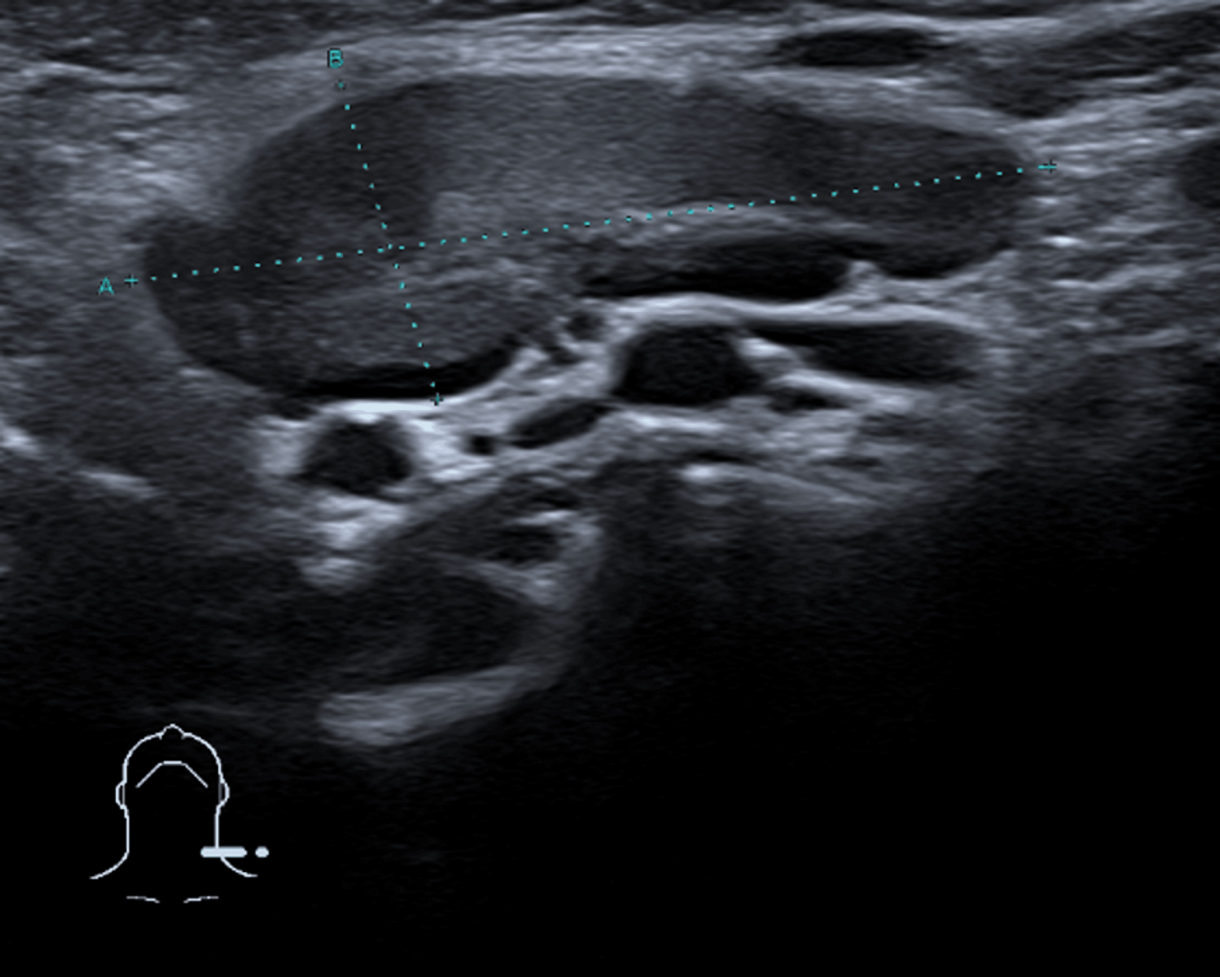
Thyroid ultrasound demonstrating a reactive left submandibular lymph node measuring 36x13 mm.
